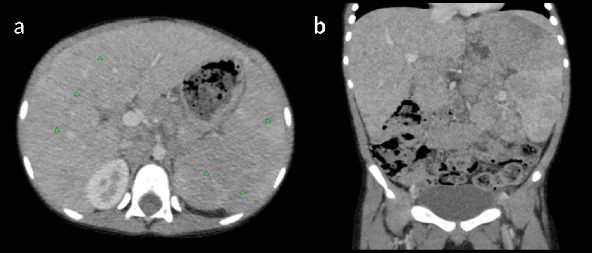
Prechemotherapy postcontrast (portal venous phase) (a) axial and reconstructed (b) coronal CT images demonstrate hepatosplenomegaly, with the liver and spleen measurements of 17.4 and 17.3 cm, respectively. Both organs show heterogeneous parenchymal enhancement. Arrowheads indicate diffuse hypoechoic parenchymal lesions within the liver and spleen.
